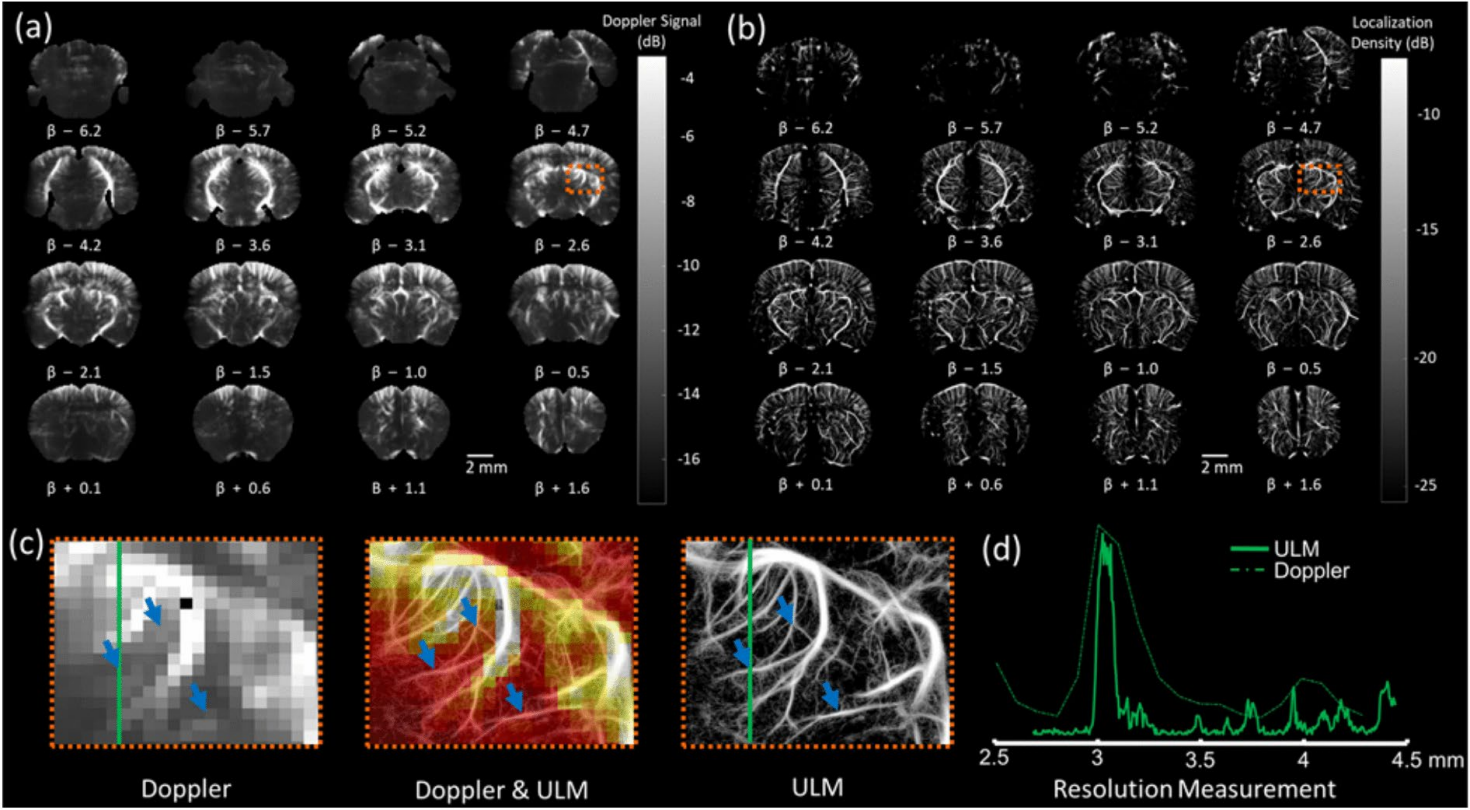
Comparison between Doppler image and ULM localization maps, covering a field of view of 11.9 × 7.87 × 8.1 mm^3^ across 16 imaging planes. β represents the z-position of each imaging plane with respect to bregma. (a) Time-averaged Doppler images highlight major vascular structures at a resolution of 110 × 98 × 525 µm. The colorbar represents the measured Doppler signal in dB. (b) Microbubble localization maps provide a more detailed representation of microvascular features at 5 × 5 × 525 µm resolution. The colorbar represents the measured MB localization signals in dB. (c) Overlaid Doppler and ULM images acquired on the same imaging plane demonstrate how ULM captures finer microvascular structures, as indicated by blue arrows. These arrows mark regions where ULM reveals microvasculature which cannot be visualized in Doppler images. (d) The intensity profile, drawn along a green line, contrasts the resolution limits of Doppler and ULM, with Doppler resolving vessels down to 234 µm and ULM detecting structures as small as 32 µm.
